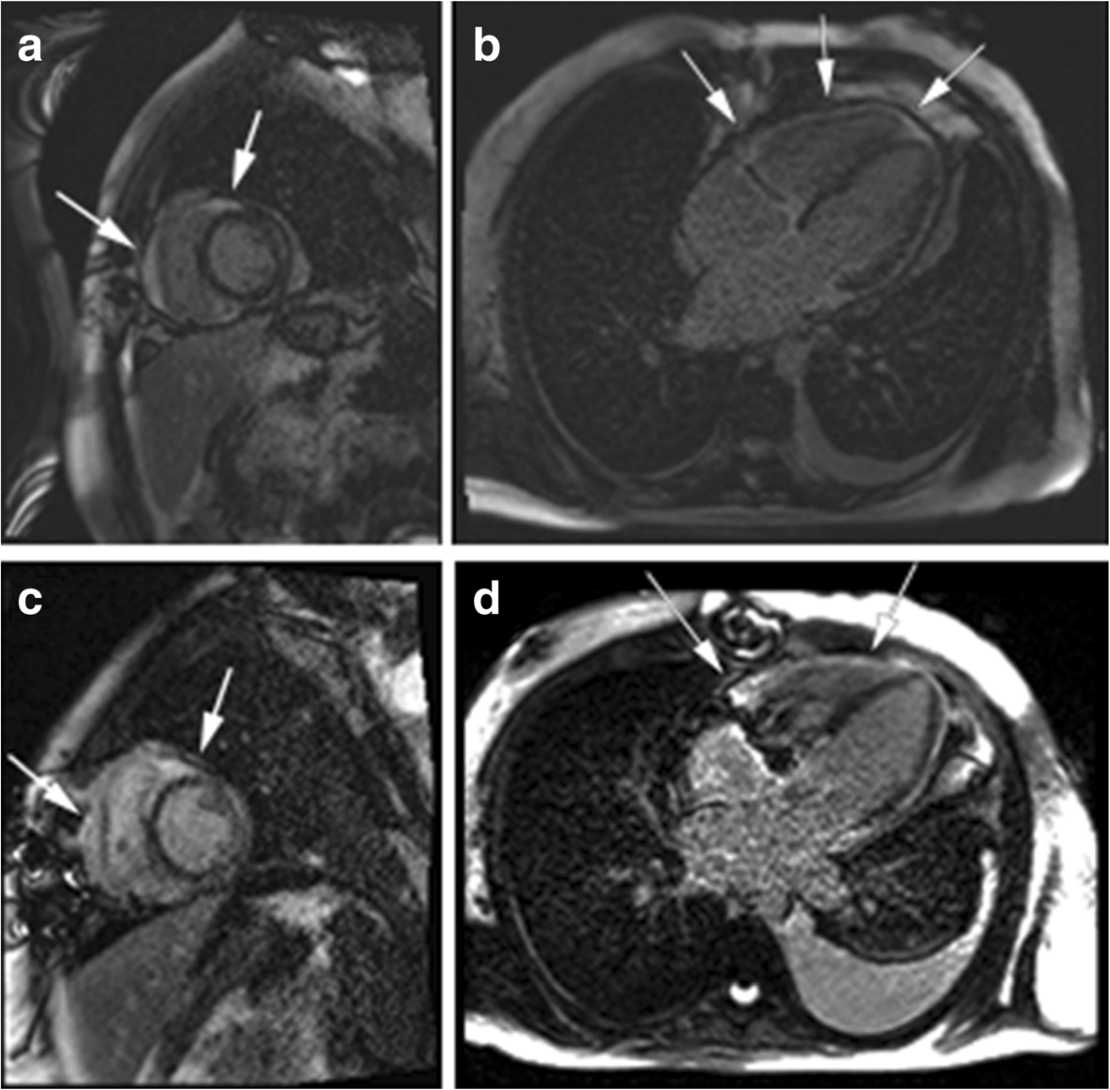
Post-contrast T1-weighted cardiac MRI images. Preoperative MRI short-axis (a) and 2-, 3-, and 4-chamber long axis (b) delayed-enhanced inversion-recovery images demonstrate pericardial thickening and epicardial enhancement (*arrows*) with subtle periventricular septal flattening. On dynamic cine imaging and anatomic imaging (not shown) there is septal bounce during Valsalva suggesting ventricular inter-dependance and biatrial enlargement with dilated IVC and hepatic veins. These findings are absent on postoperative cardiac MRI (c-d)
